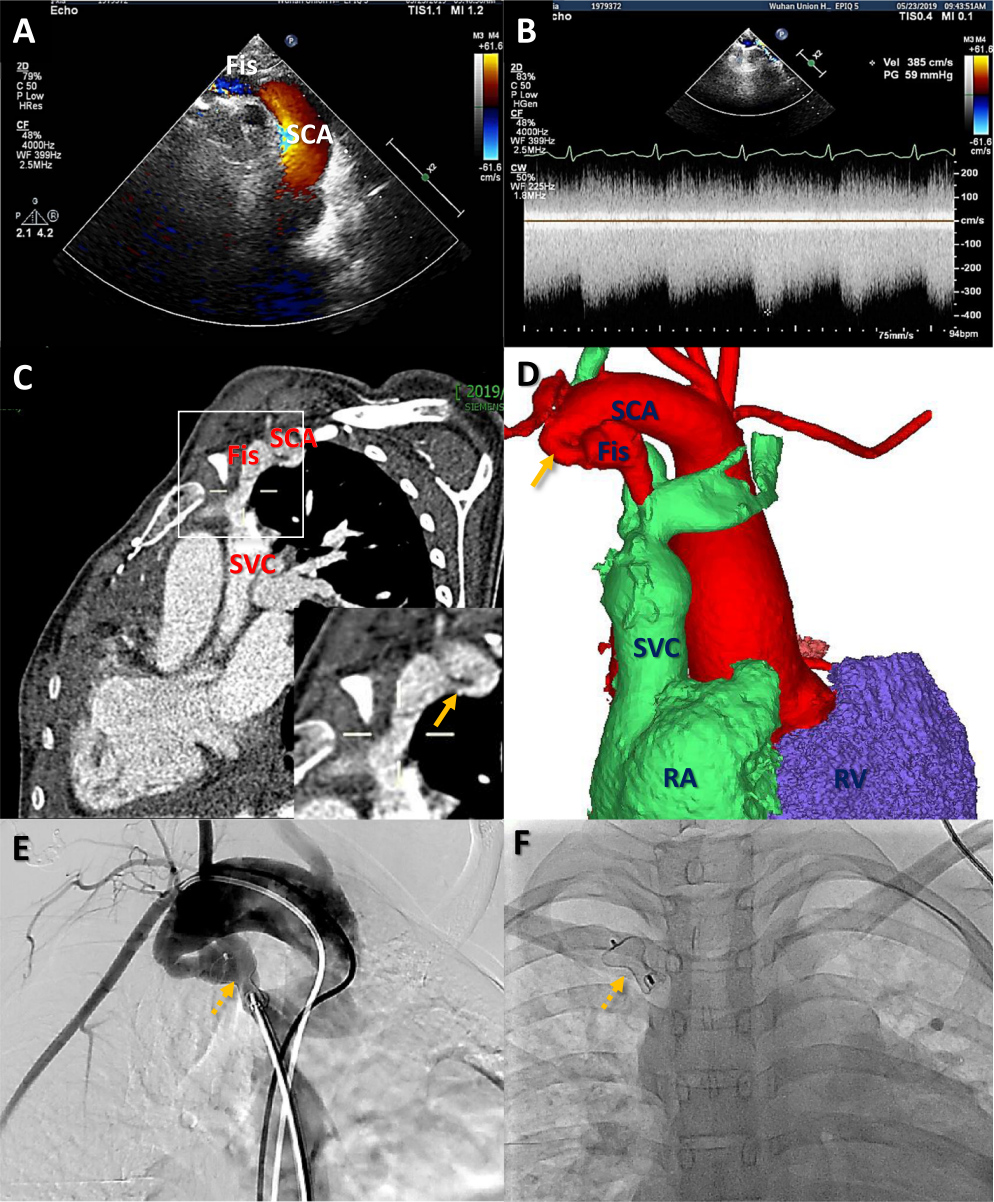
a Transthoracic echocardiography shows the arteriovenous fistula (Fis) between the right subclavian artery (SCA) and the superior vena cava (SVC); b Continuous-wave Color Doppler interrogation of the fistula shows high velocity flow signals moving from the SCA into the SVC throughout the cardiac cycle (stenosis site: velocity 385 cm/s, pressure gradient 59 mmHg); c, d Computed tomographic angiography and three-dimensional reconstruction show the fistula and obvious stenosis site (arrow); e,f The fistula is successfully closed using a 10/12 mm Amplatzer Ductal Occluder (asterisk) by the guidance of angiography (dotted arrow); RA — right atrium; RV — right ventricle
